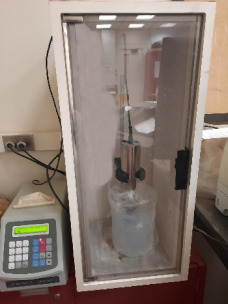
Qsonica	Newtown, CT,USA	Q-700
